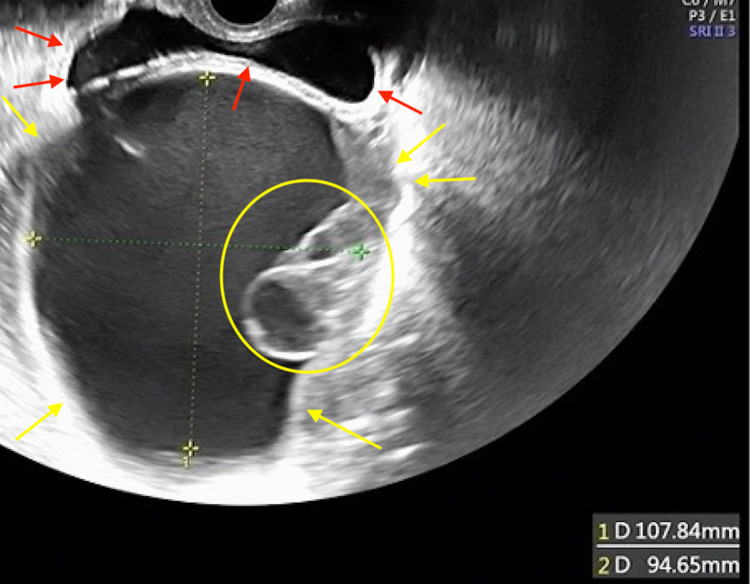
Transvaginal ultrasound image of the ovarian tumor Transvaginal ultrasound showing a 10-cm-large monoblastic ovarian tumor Yellow arrows: ovarian tumor; yellow circle: cystic component; red arrows: cyst
